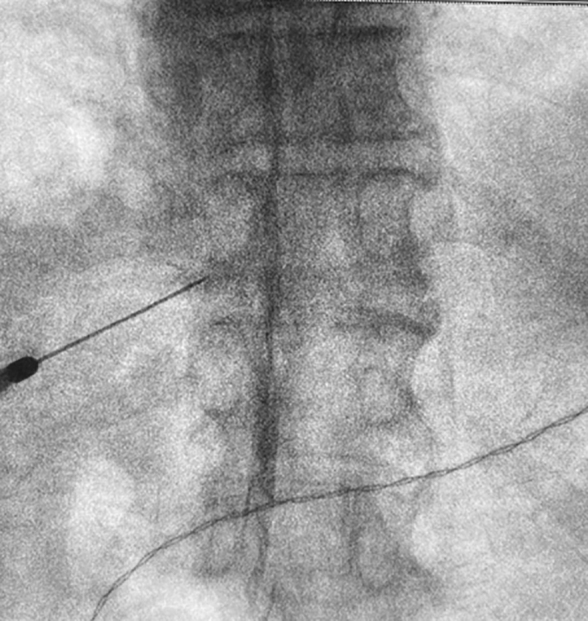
X-ray image of the thoracic spine. Radiofrequency thermocoagulation is performed under fluoroscopic guidance.
